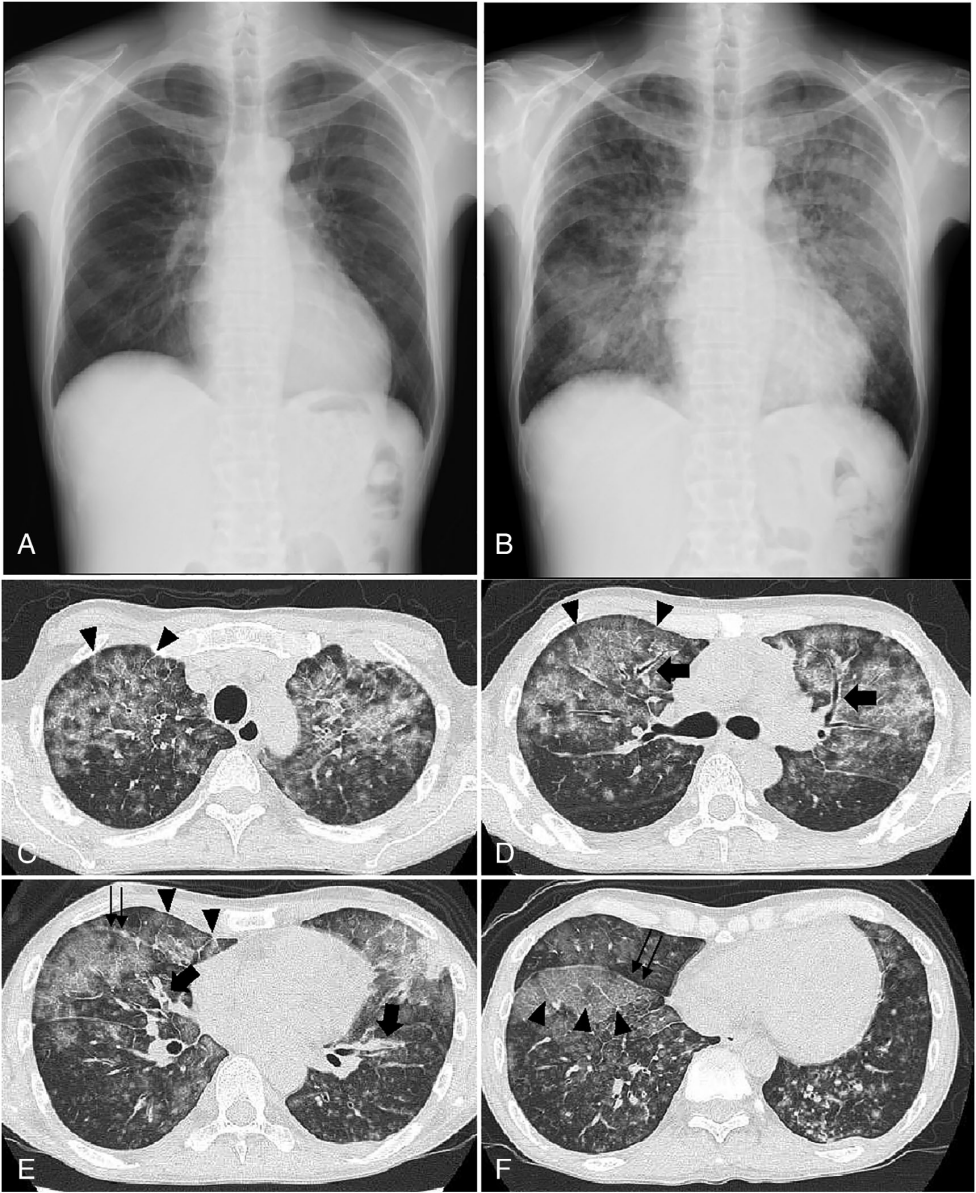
Normal chest X‐ray findings one month before admission to our hospital (A). Diffuse pulmonary infiltrates was noted in both lung fields at the time of admission (B). Non‐enhanced thoracic computed tomography (CT) on admission demonstrated ground‐glass opacity in both the lungs (C–F) with thickening of broncho‐vascular bundles (D, E; arrows), interlobular septa (C–F; arrow heads) and right interlobar fissure (D, E; double arrow). Randomly distributed tiny nodules were predominantly seen in both the lower lungs (E, F).
